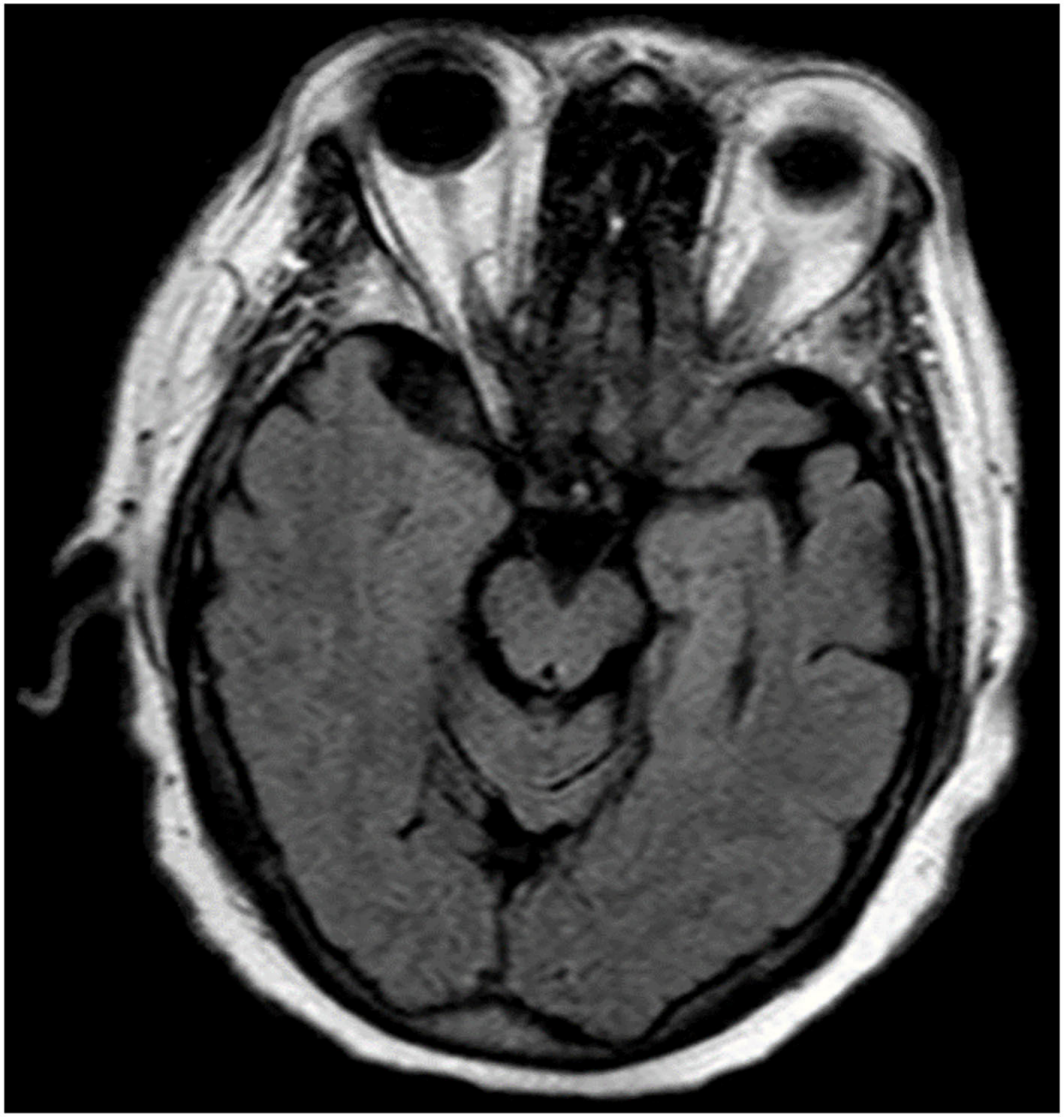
The patient's magnetic resonance imaging revealed low brain volumes in the anterior temporal lobe and frontal lobes.
